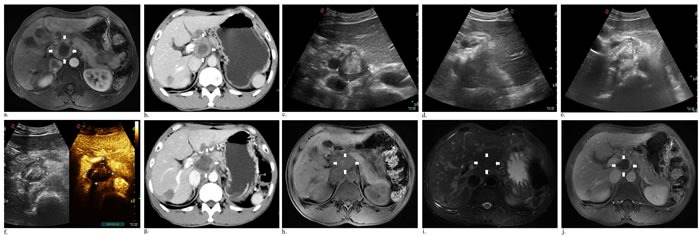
Axial abdominal MR image performed 13 days after initial ablation revealed the peripheral remanent tumor a. (white arrows), 6-week follow-up CT scan of the venous phase measuring 4*3.1 cm with area of central necrosis 2.3*1.8 cm b. (white arrows). Before the second laser ablation, a central well-defined hyperecho surrounding unenhanced hypoechoic active areas c.. During ablation, immediate US scan showed the left part of tumor obvious enhancement d., and the next day a finding that most response appeared e., f.. At a follow-up visit 11 days, a contrast-enhanced CT venous phase image revealed the enlarged areas of tumor necrosis g. (white arrows). Then two months later, there was reduced mass necrosis of 2.43*1.7 cm at T1 h. (white arrows), T2 i. (white arrows) and substance phase j. (white arrows) of MR image.
